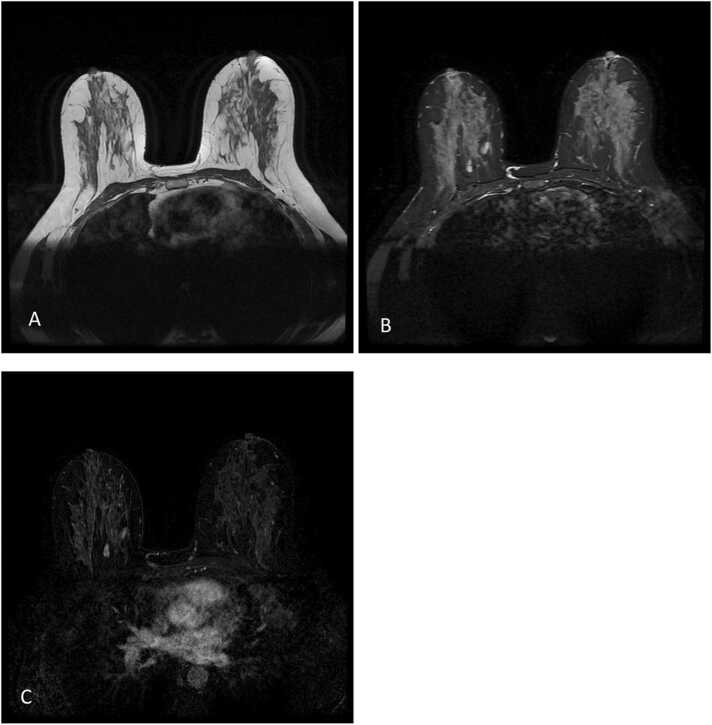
Axial T1-weighted (A), Axial T2-weighted (B) and Axial post contrast T1-weighted (C). Images show circumscribed persistent enhancing mass in right breast deep central zone.
